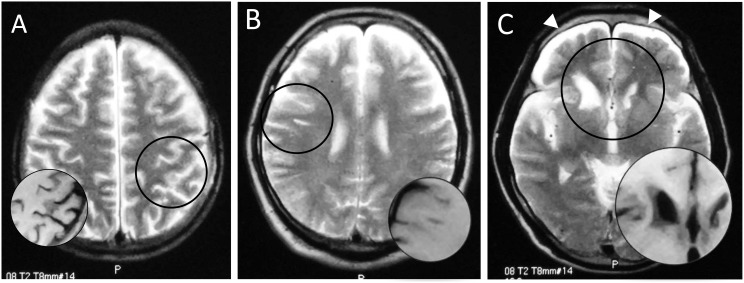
Magnetic resonance images of brains of three Ama divers: hyperintense area on T2-weighted image (circle), corresponding to hypointensity on T1-weighted image (inset). A patchy shadow in the left parietal cortex (A, No. 2), a linear subcortical lesion in the right frontal lobe (B, No. 5), and deformity of bilateral caudate heads and subdural fluid collection (allow heads) (C, No. 11).
